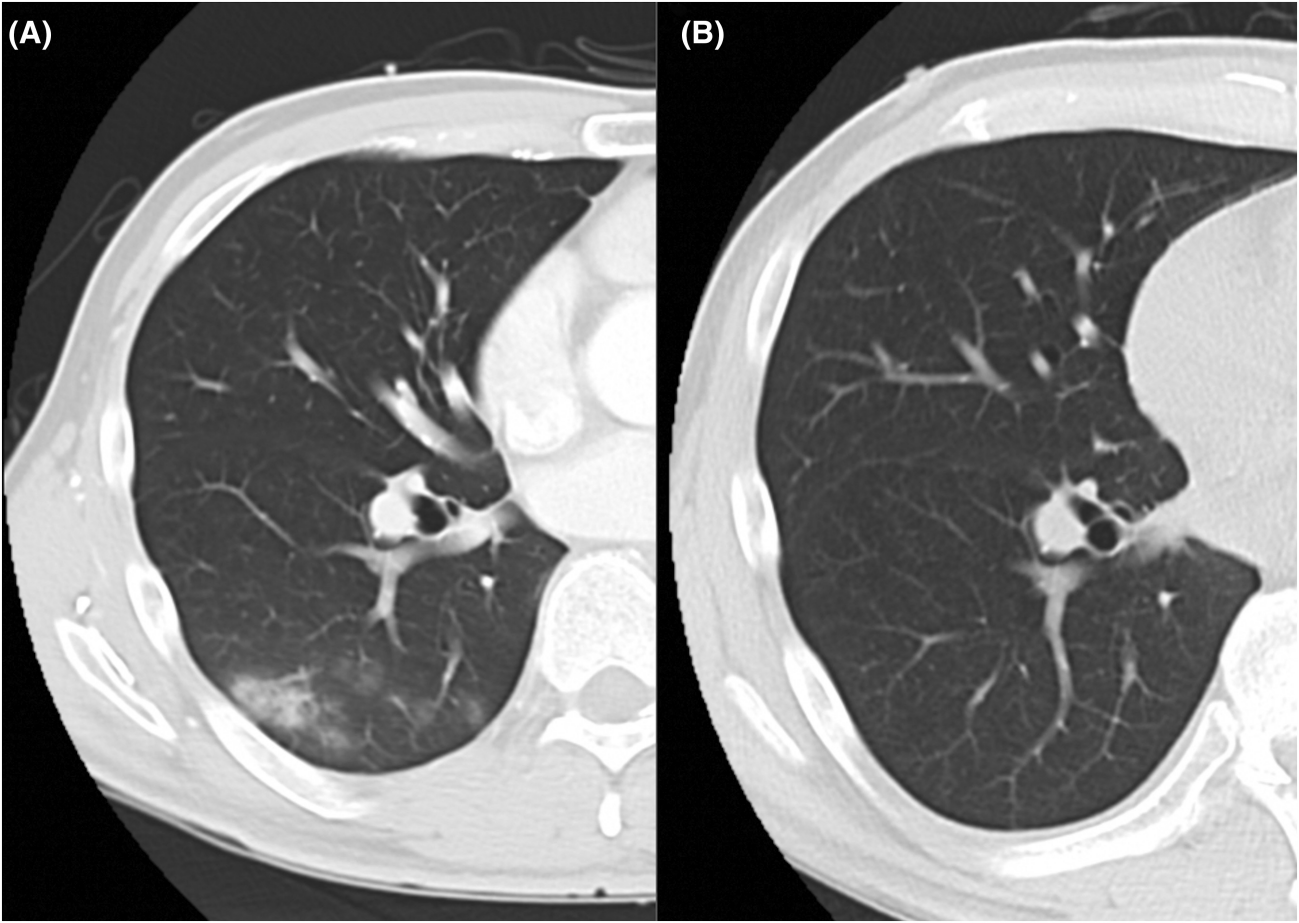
(A) Chest CT shows pulmonary opacity localized only in the superior segment of the right lower lobe. (B) On postoperative chest CT, no opacity was revealed.
